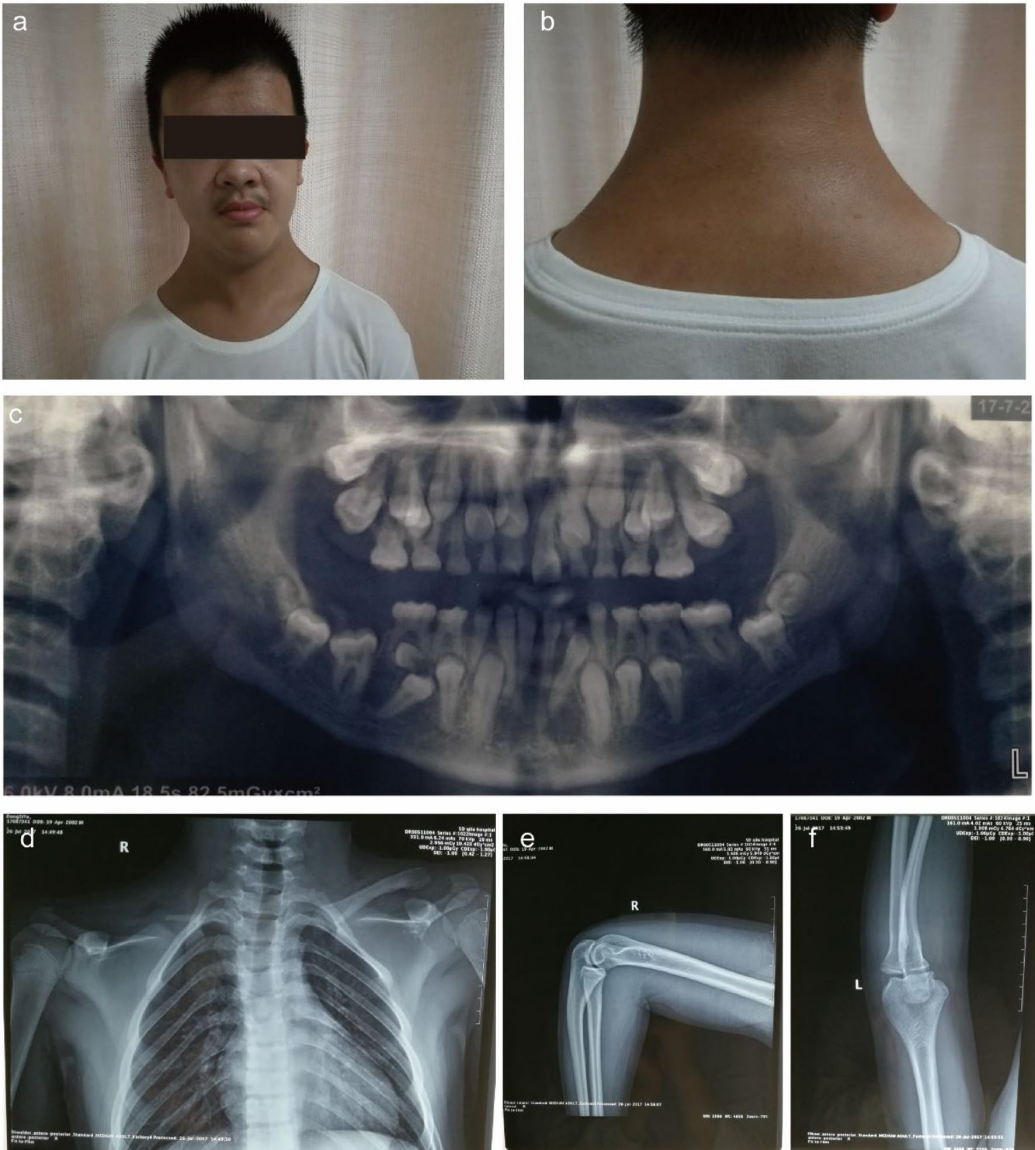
Typical and radiological findings in the CCD patient. a Frontal facial view of patient representing midline depression of forehead and bilateral hypoplastic clavicles. b Hypoplasia of the clavicles abnormal facility in the opposing shoulders. c Panoramic radiography revealed primary teeth retention, numerous impacted permanent teeth in both maxilla and mandible. d Chest X-ray showed bilateral hypoplastic clavicles hypoplasia of iliac bones, wide symphysis pubis with a bell-shaped thoracic cavity and scoliosis. e, f No abnormalities were seen in the bones of the bilateral elbow joints, and the epiphyseal line showed closure
